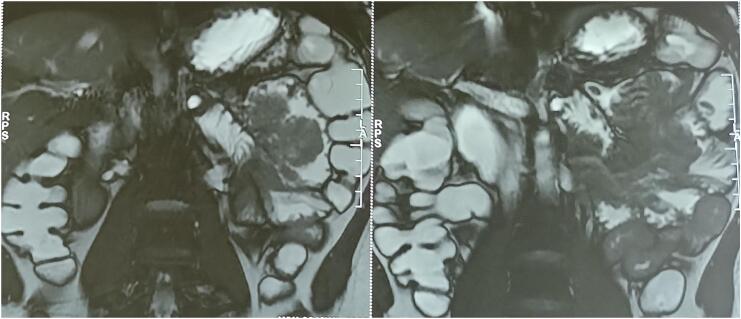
Entero-MRI showing a jejuno-jejunal intussusception associated with a voluminous duodenal polyp,7 cm wide with a large pedicle.
